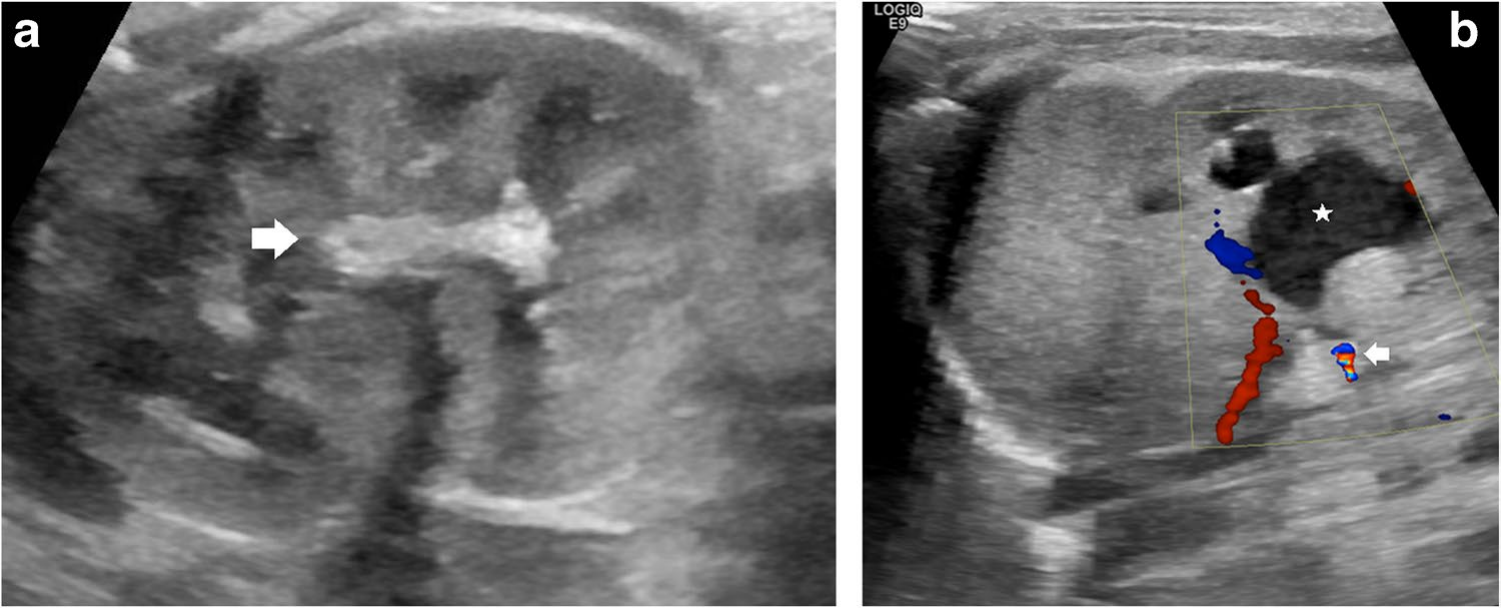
Drug-induced urolithiasis (post-diuretic) in a premature 35-week-old boy (born at 26 weeks of gestational age). a Sagittal US of the left kidney shows a large, calcified structure in the renal hilum (*arrow*), corresponding to a stone. b Follow-up US colour Doppler examination after 5 weeks. Transverse view of the kidney shows the stone to have dissolved, with formation of a calculus in the proximal part of the ureter visualised by virtue of the twinkling artifact (*arrow*). Obstruction of the ureter resulted in pelvicalyceal dilatation with echogenic concrement in the renal pelvis (*asterisk*). Case courtesy of Prof. Philippe Petit, Marseille, France
